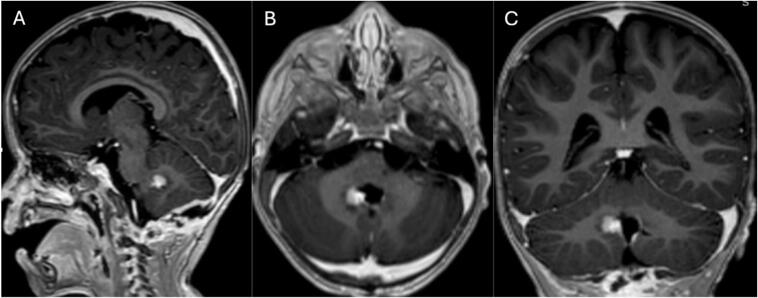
Magnetic resonance imaging of the brain: first imaging after chemotherapy initiation post-surgical intervention.
